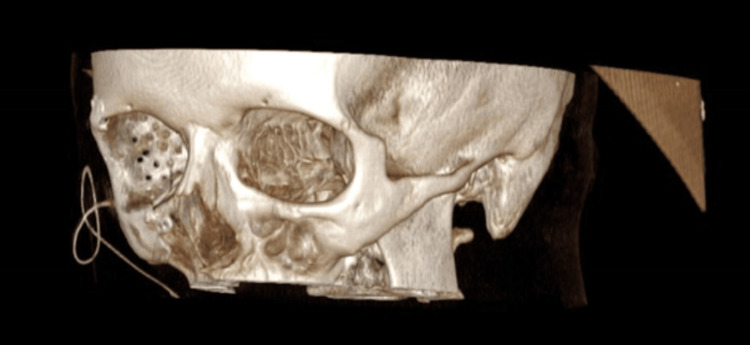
3D left-sided view of CT head with right orbito-zygomatic implant
